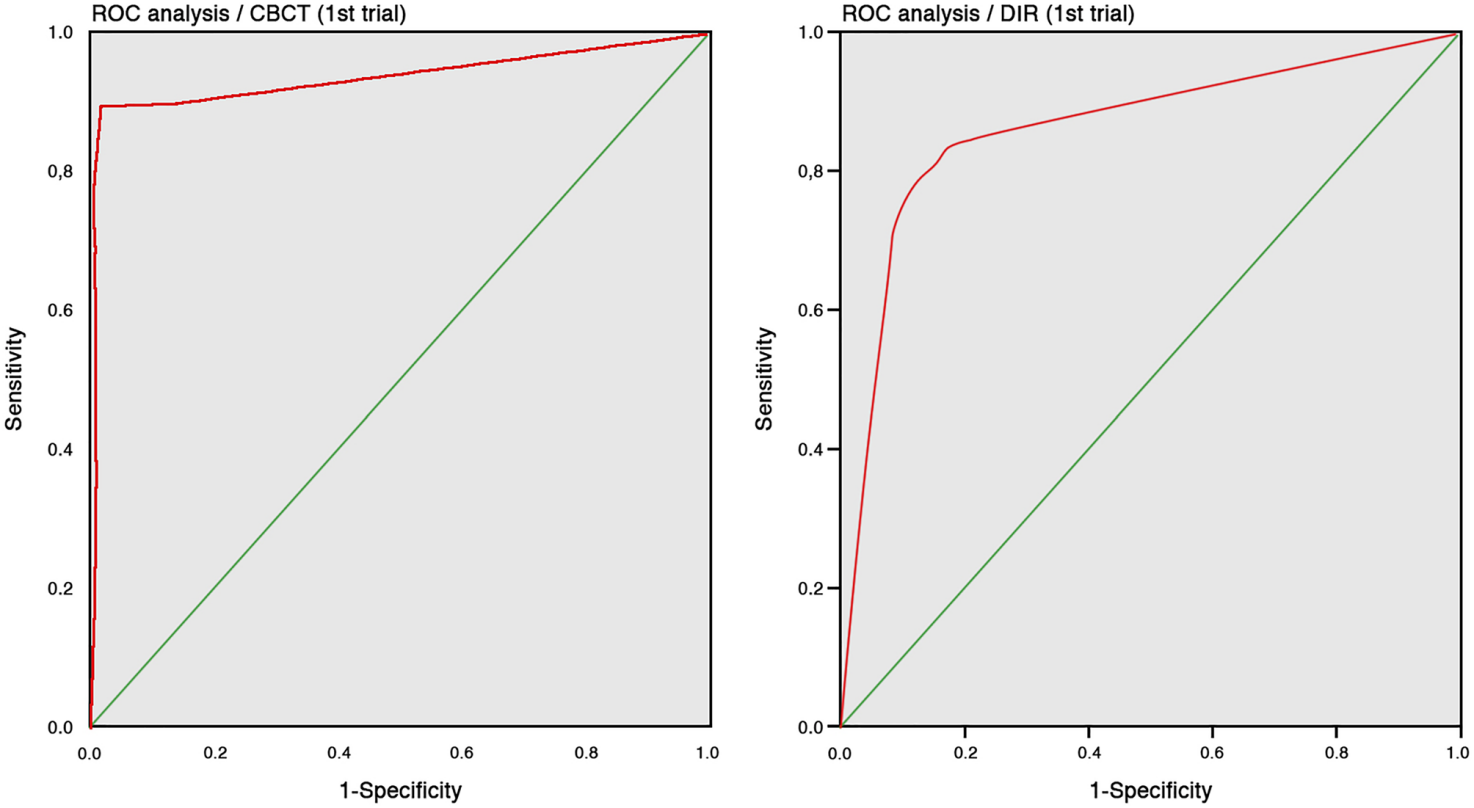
ROC analysis: CBCT (left) and DIR (right) of the first trial being the16 observers pooled with both image acquisition methods.
